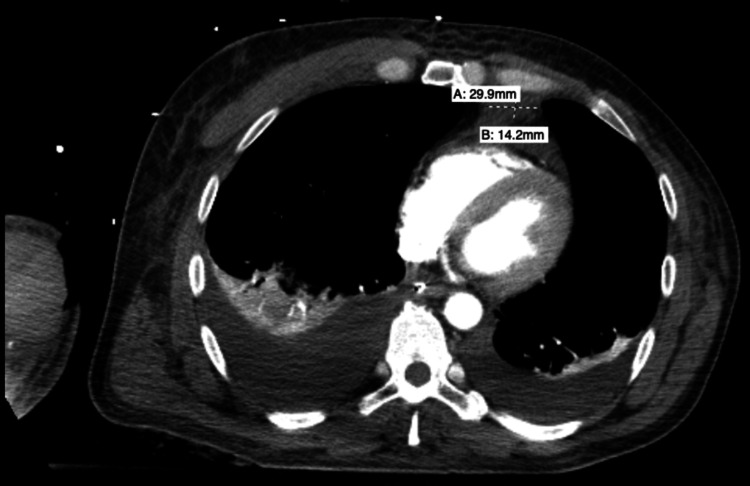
CT of the chest demonstrating a soft tissue nodular density in the anterior mediastinum measuring 3.0 cm x 1.4 cm, suggesting possible mediastinitis.
